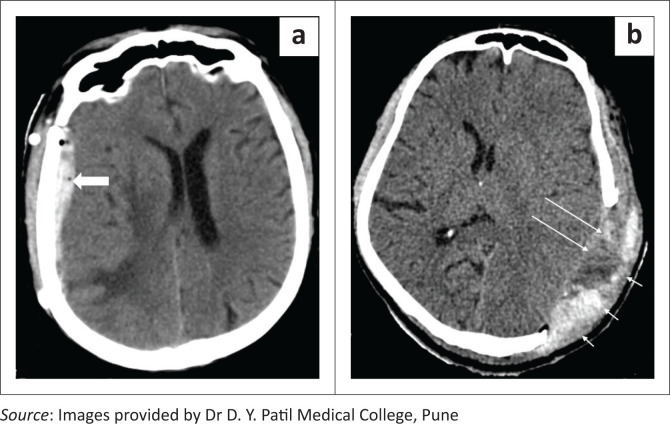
Axial soft tissue window CT image (a) in a patient with a right fronto-parietal craniotomy demonstrates a lentiform hyperdense extra-axial hematoma (thick white arrow) in the right fronto-parietal region with mass effect causing effacement of the right lateral ventricle and midline shift toward left side. Axial soft tissue window CT image (b) in another patient with a left parietal craniectomy reveals a lentiform mixed attenuation epidural hematoma (long arrows) and subgaleal hematoma (short arrows) at the craniectomy site, causing mass effect and resultant effacement of the left cerebral sulci and lateral ventricle with midline shift to right side.
